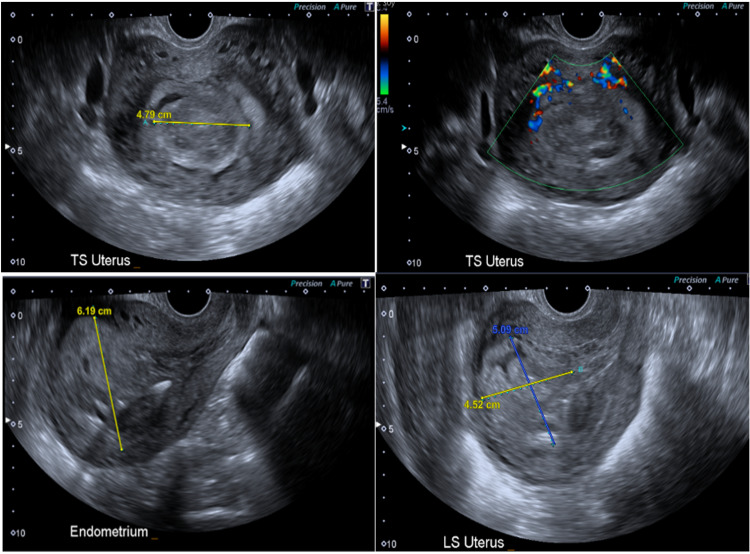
Sonographic findings. TS: transverse section; LS: longitudinal section.
